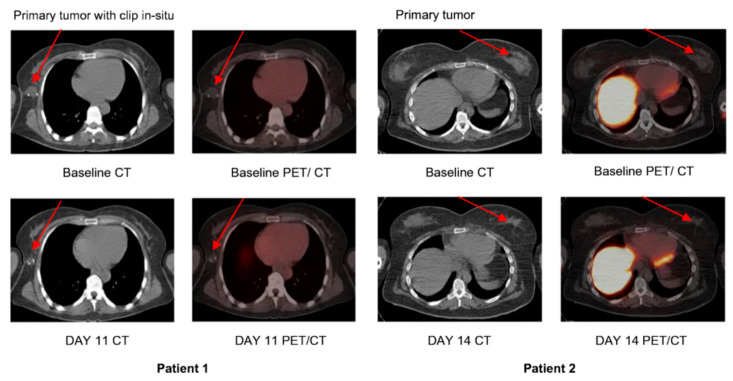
[^18^F]ICMT-11 uptake in primary breast tumors. Axial CT and fused [^18^F]ICMT-11 PET/CT images of primary breast tumors in two patients, 1 and 2, at baseline (pre-) and post-chemotherapy. Low-level uptake is noted. Reprinted with permission from ref. [35]. Copyright 2018 Springer Nature.
